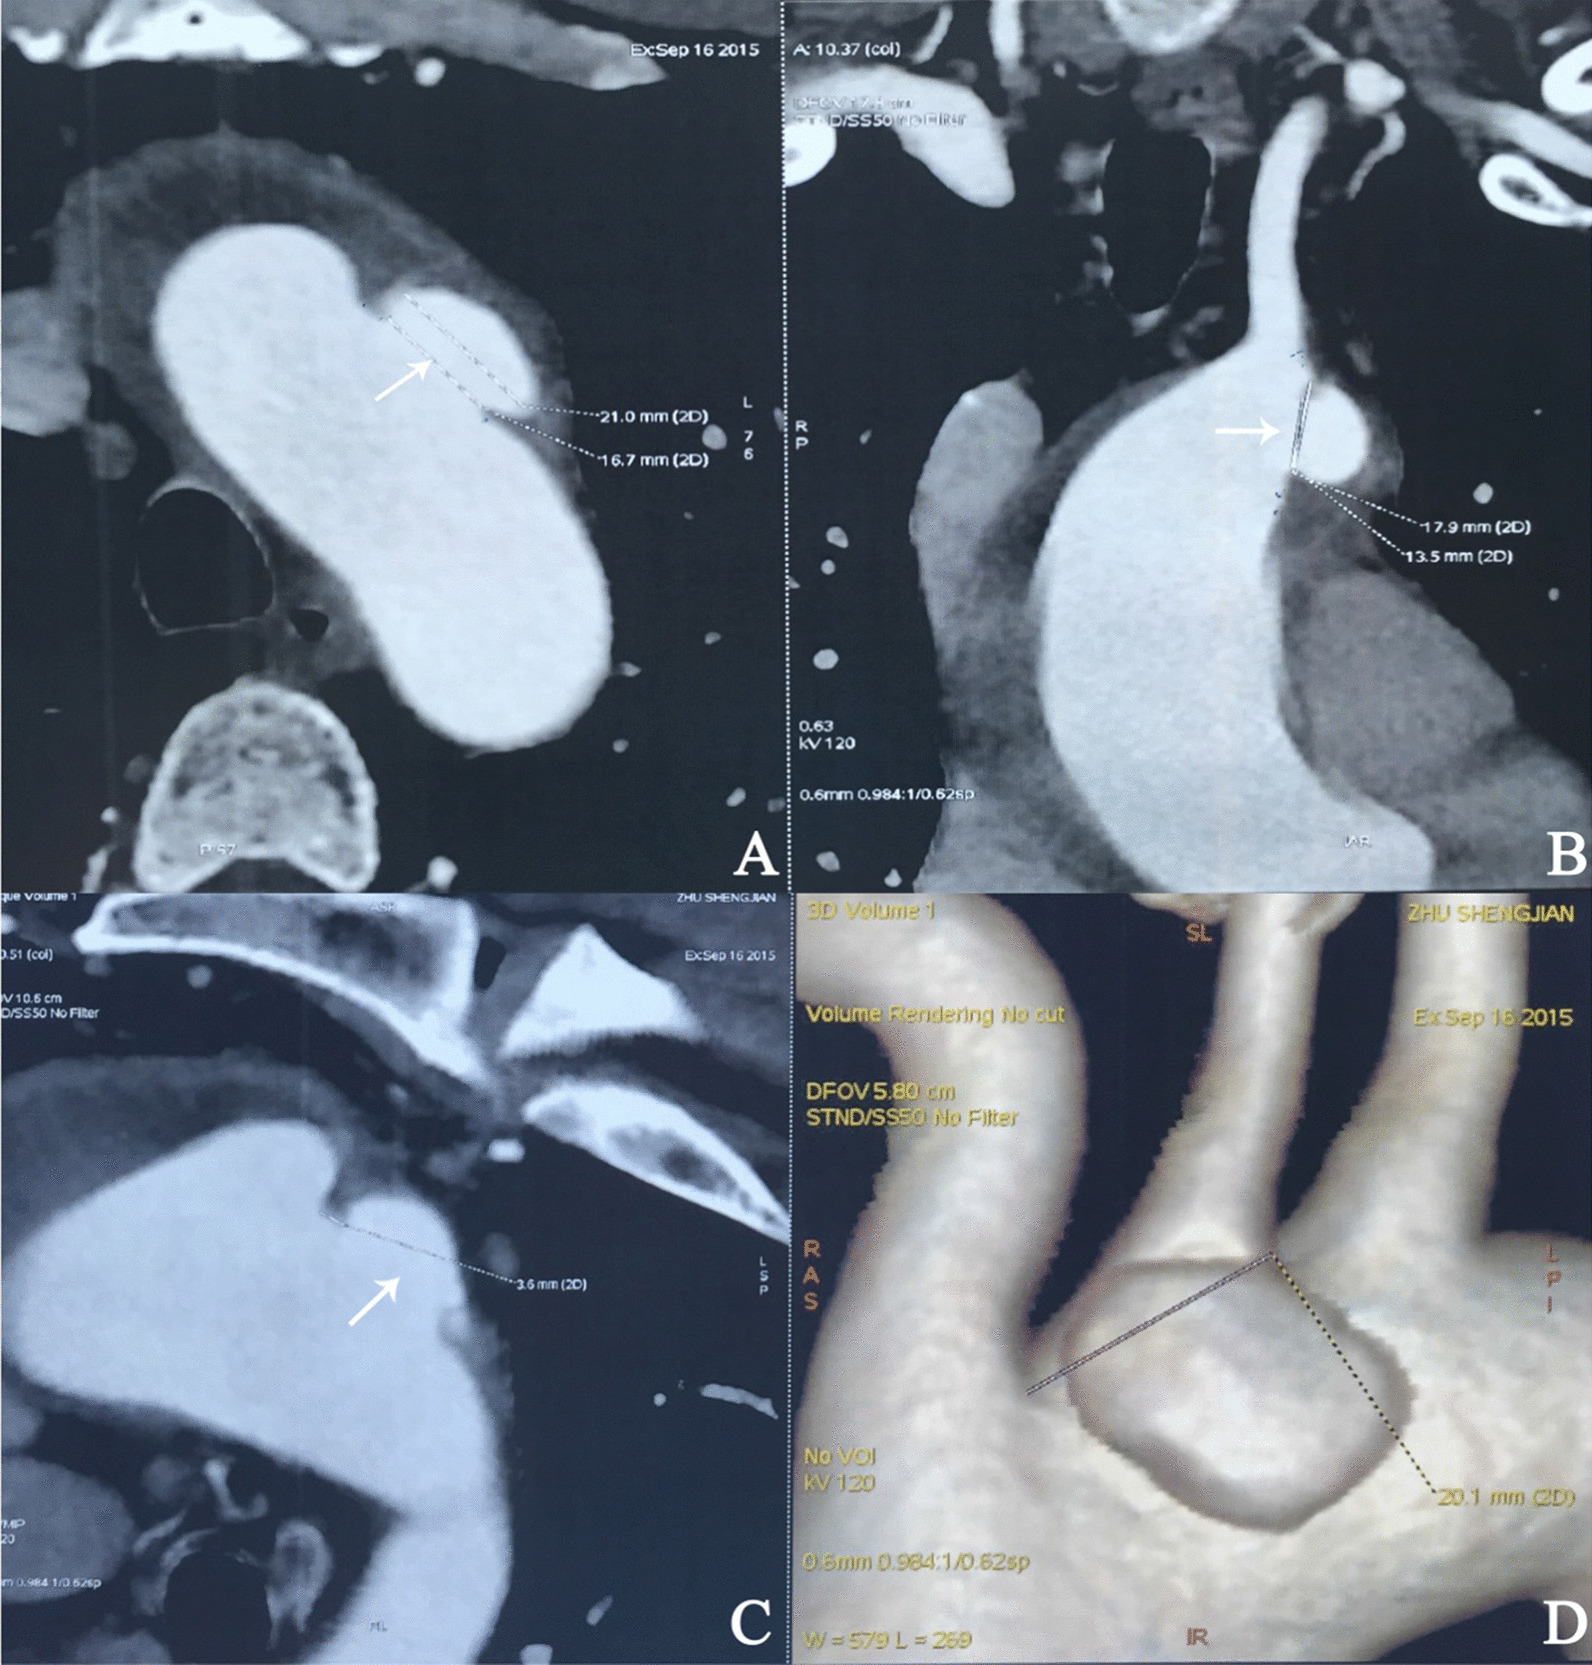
Preoperative imaging. Computed tomographic angiography (CTA) showing a penetrating aortic ulcer (PAU) that occurred in the aortic arch, with an intramural haematoma (IMH) compromising the ascending aorta and aortic arch
